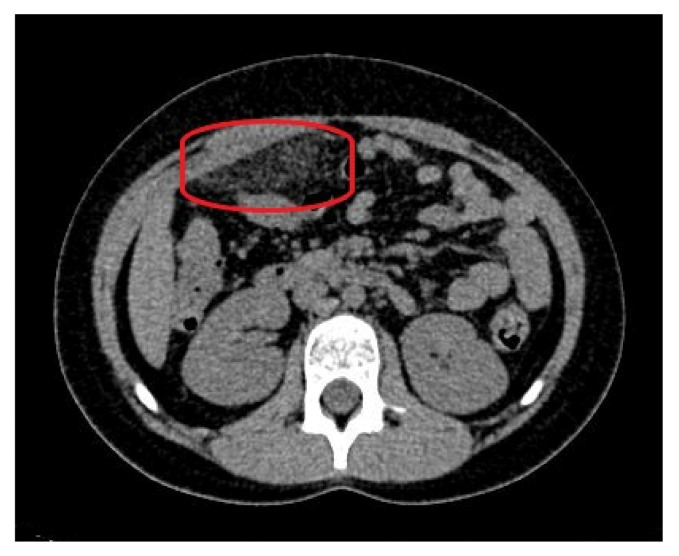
The computed tomography of the abdomen on admission: fat stranding in the right epigastrium (marked with a red ellipse).
